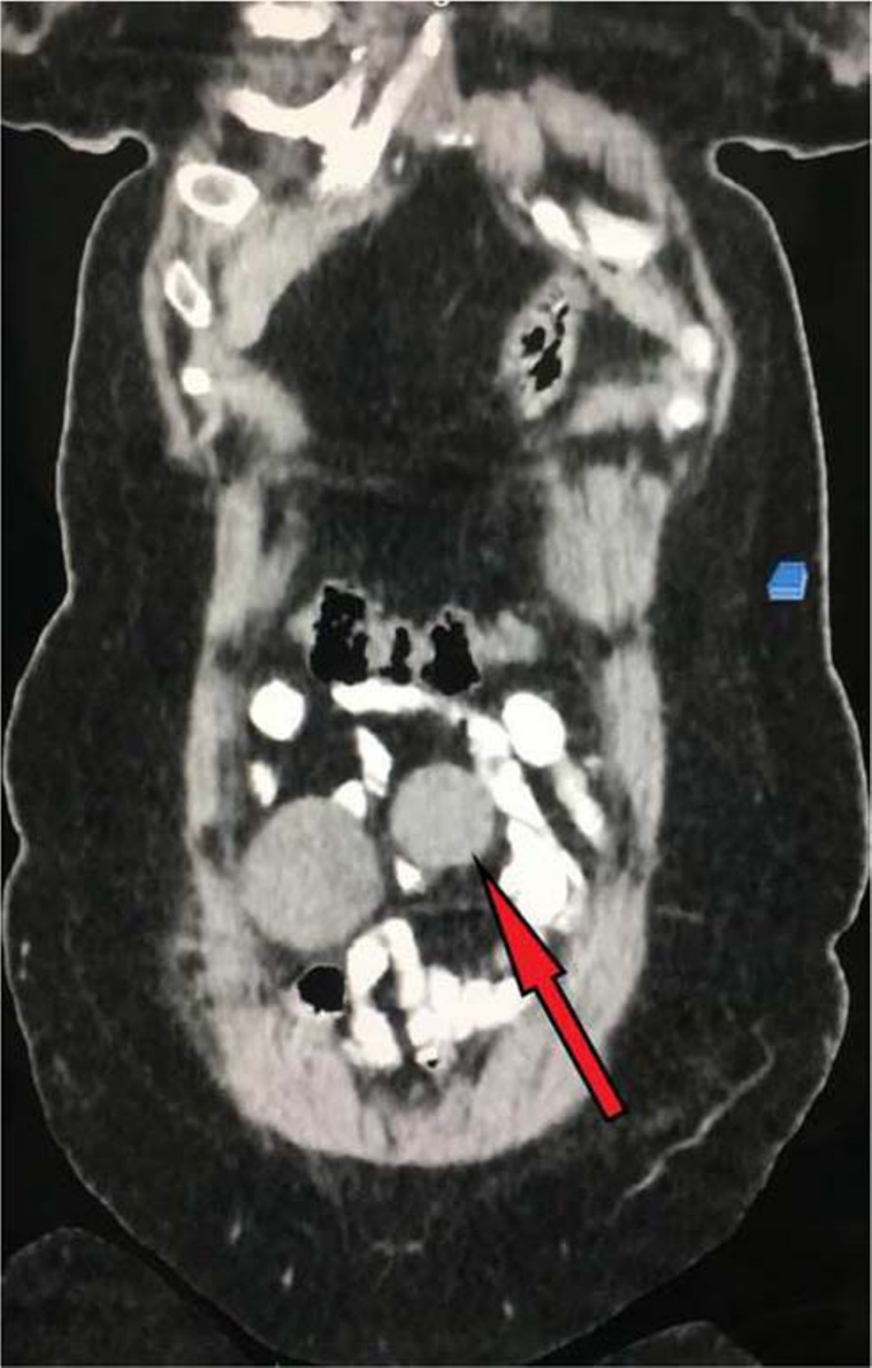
CT scan image shows a dumbbell-shaped tumor (marked by red arrow) attached to the small intestine
